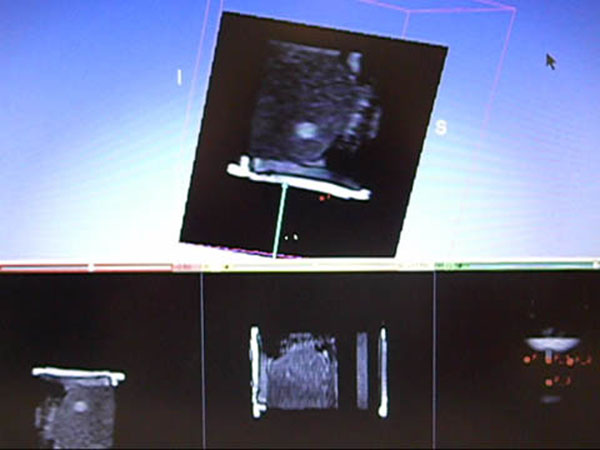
3D slicer image of MRI with high intensity area was displayed in a manner resembling conventional monitor US to assist the FUS treatment.
